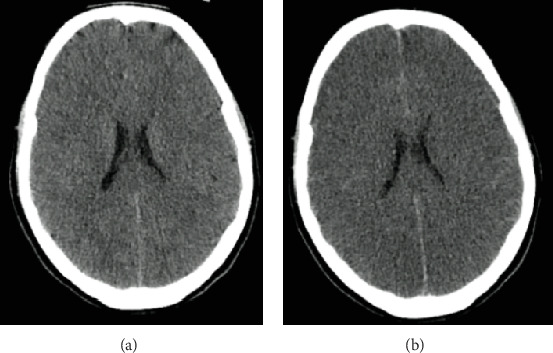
CT head scan at admission to the hospital (a) and at 48 h (b). On the right, the image shows diffuse hypodensity of cerebral hemispheres suggestive of ischemia as well as sulci loss and ventricular system reduction indicative of diffuse cerebral oedema.
